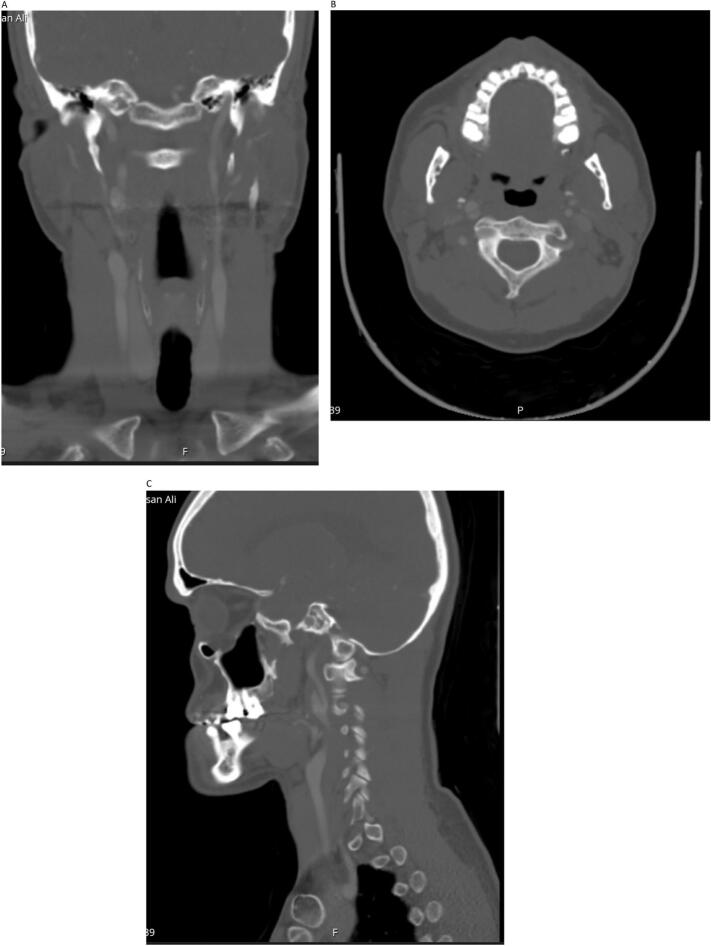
A: CTA coronal reconstruction showing shrinking right ICA pseudoaneurysm. B: CTA right ICA dissection. C: CTA sagittal view right ICA pseudoaneurysm with thrombosis.
